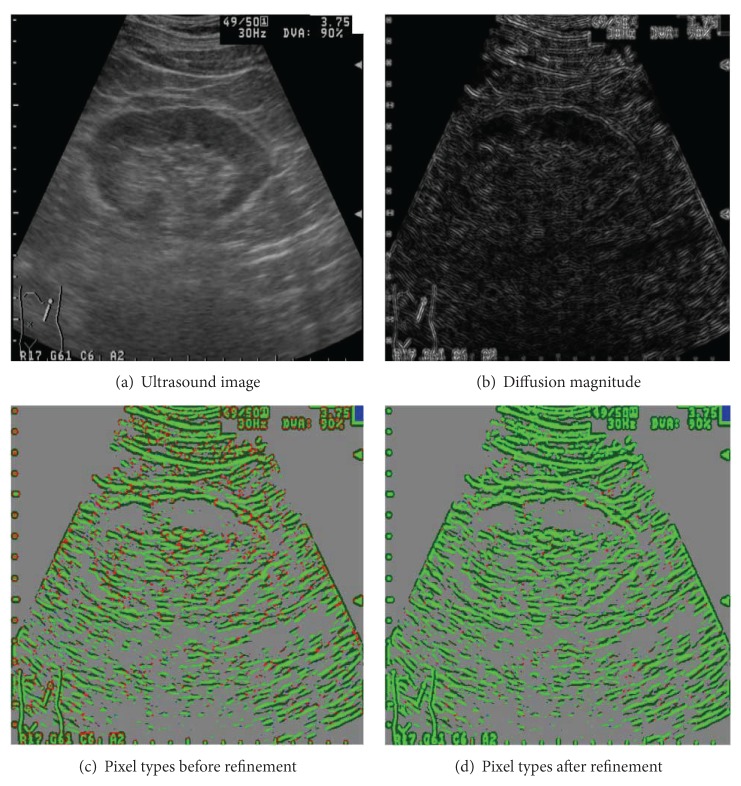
Structure type classification, (a) the ultrasound image, (b) the diffusion strength, (c) classified structure types, and (d) refined structure classification, green: linear, dark green: boundary, red: spot, blue: unknown, and grey: uniform.
